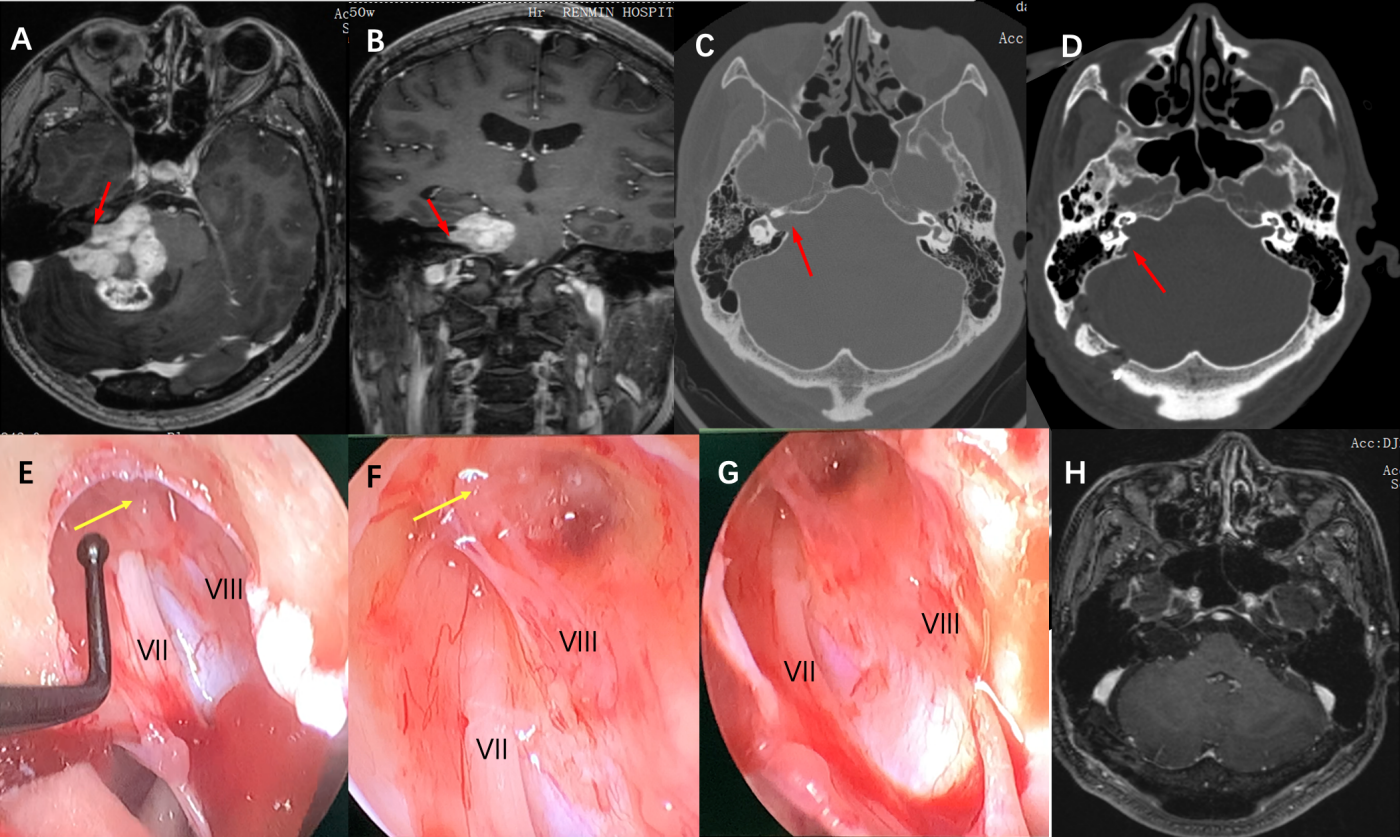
Imaging studies and intraoperative snapshots of a case of Grade IV VS extending into the internal auditory canal. (A) The preoperative T1 contrast image revealed a giant VS at the CPA area. The intracanalicular portion of the tumor was irregularly shaped as indicated by the red arrow (axial plane). (B) giant VA abutting the brainstem and extension into the internal auditory canal as indicated by the red arrow (coronal plane) (C) Thin-section CT scan of the skull base showed that the ipsilateral internal auditory canal was significantly enlarged compared with the contralateral side (red arrow), and the mastoid air cells were well gasified. (D) A postoperative CT scan showed that the posterolateral part of the internal auditory canal bone was removed (red arrow), and the structure of the bony labyrinth and semicircular canal was intact. (E) Intra-operative neuroendoscopy view of the operation showed that after the tumor was removed in the CPA area, the internal auditory canal was opened and there were tumor remnants in the internal auditory canal (yellow arrow), and nerves VII and VIII are located anterior and posterior inferior to the internal auditory canal respectively. (F) Intraoperative neuroendoscopy view showed after tumor resection, there were still tumor remnants at the bottom of the internal auditory canal adhering to the transverse crest (yellow arrow). (G) Intraoperative neuroendoscopy showed that after complete resection of the tumor in the internal auditory canal, the bottom structure of the internal auditory canal showed, and the VII and VIII nerves were intact. (H) Postoperative MRI review after 1 year showed complete tumor resection and no tumor recurrence.
